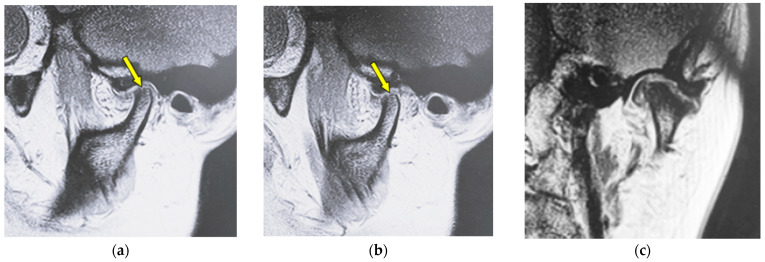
MR image (proton-density-weighted) of a 43-year-old woman (disc displacement without reduction, left disc): (a) sagittal oblique cross-section imaging in the mouth-closing position; (b) sagittal oblique cross-section imaging in the mouth-opening position; (c) coronal oblique cross-section imaging in the mouth-closing position. The arrow shows the part with erosion.
